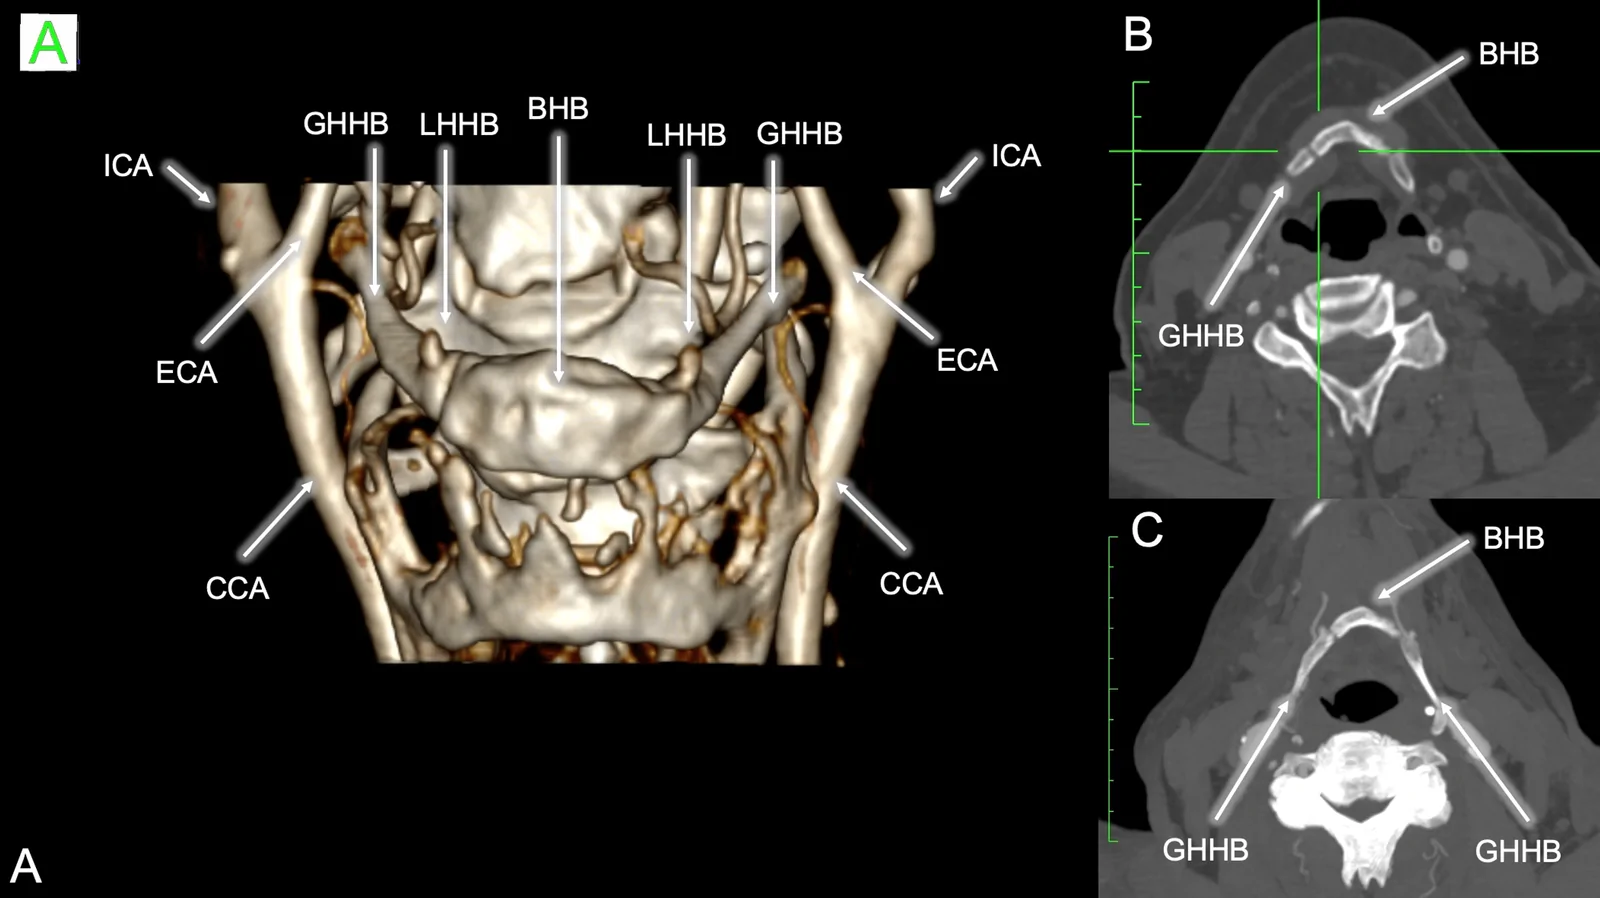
Computed tomography angiography of a 53-year-old man showing asymmetric body–greater cornu fusion, with persistence of the right body–greater cornu junction. (A) Anterior three-dimensional volume-rendered reconstruction showing the hyoid bone and its relationship to the carotid arteries. (B) Axial source image demonstrating the persistent right-sided body–greater cornu interface. (C) Axial maximum-intensity-projection reconstruction showing the hyoid body and both greater cornua. BHB, body of the hyoid bone; CCA, common carotid artery; ECA, external carotid artery; GHHB, greater cornu of the hyoid bone; ICA, internal carotid artery; LHHB, lesser cornu of the hyoid bone. Image Credit: Dr. George Triantafyllou.
